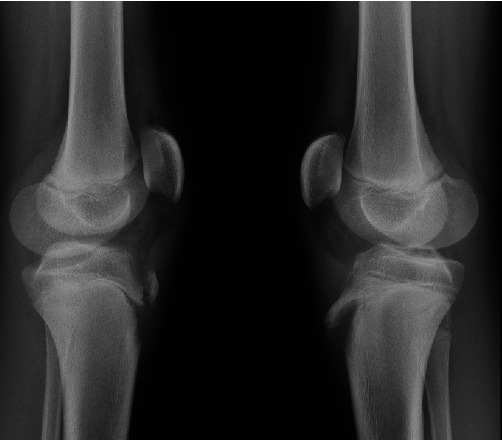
Case II: X-ray imaging asymmetrical avulsion fractures with a type IIA of the right knee and type II B of the left, again without intra-articular involvement.
